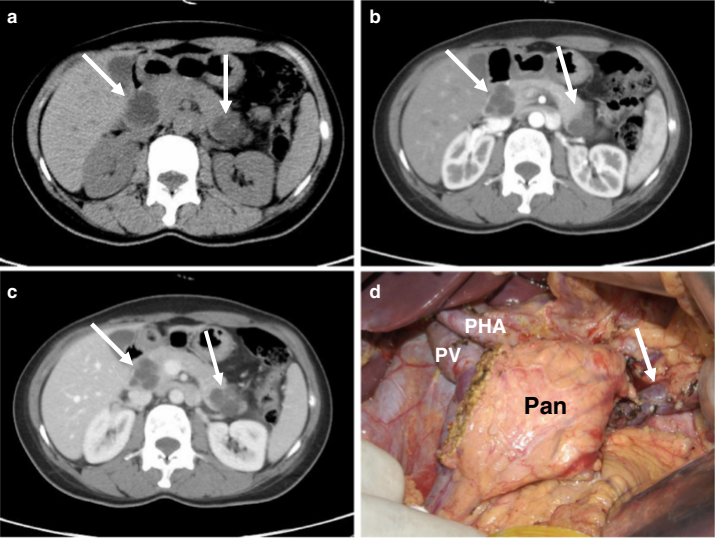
CT scans and intraoperative imaging of patient two. a) Plain CT revealed two well-defined cystic lesions (white arrow). b) Enhanced CT demonstrated polycystic appearance of the tumor with no enhancement in the arterial phase (white arrow). c) Enhanced CT demonstrated polycystic appearance of the tumor with no enhancement in portal phase (white arrow). d) After a simultaneous standard PD and spleen-preserving atypical LP, about 5 cm of the pancreatic body (Pan) and the splenic vein (arrow) were preserved. Pan, pancreas; PHA, proper hepatic artery; PV, portal vein.
